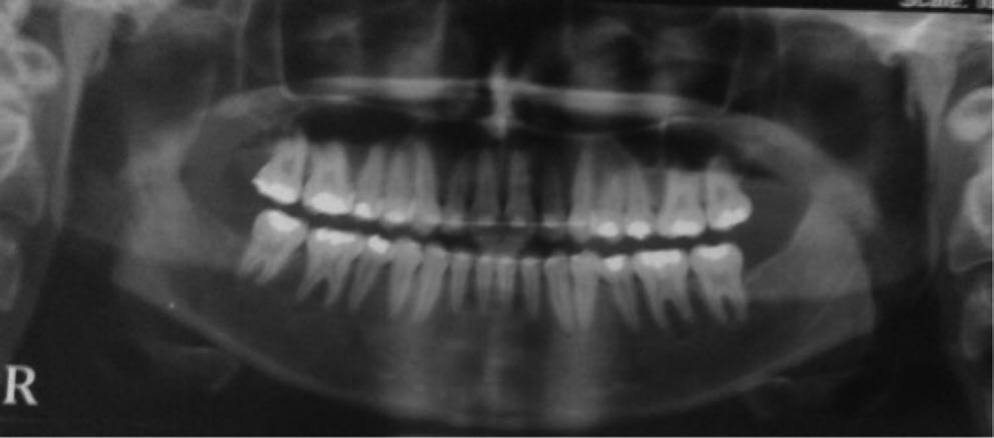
One‐year postoperative radiograph
